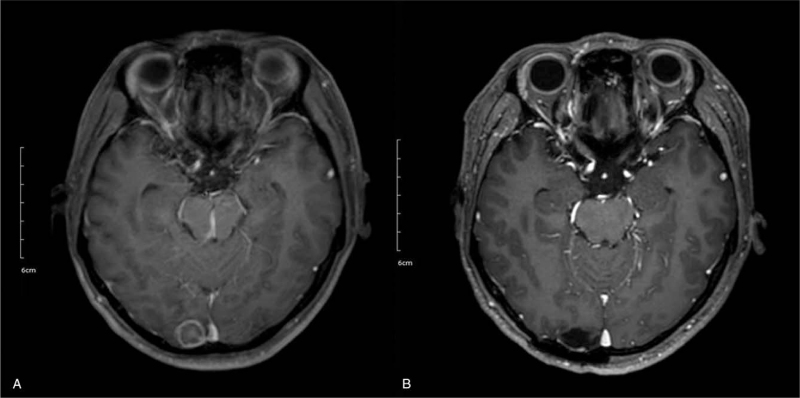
(A) Brain MRI shows 1.5 cm sized rim enhancing mass in the right occipital lobe. (B) About 5 mo after mass removal, brain MRI shows no definite residual lesion in the right occipital lobe. MRI = magnetic resonance imaging.
